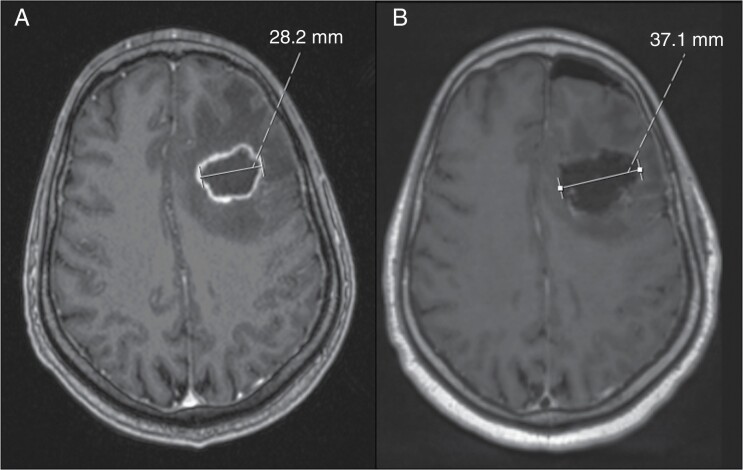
(A) Pre- and (B) postoperative axial contrast-enhanced MRI showing the size of the resection cavity beyond the limits of contrast enhancement.
